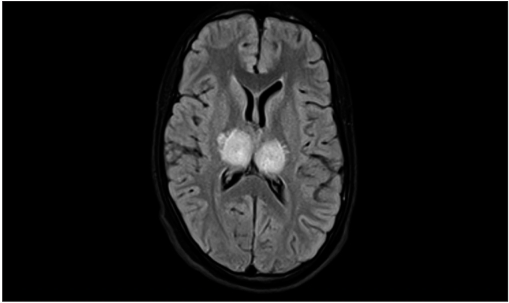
MRI T2-weighted FLAIR image showing bilateral thalamic hyperintensity
